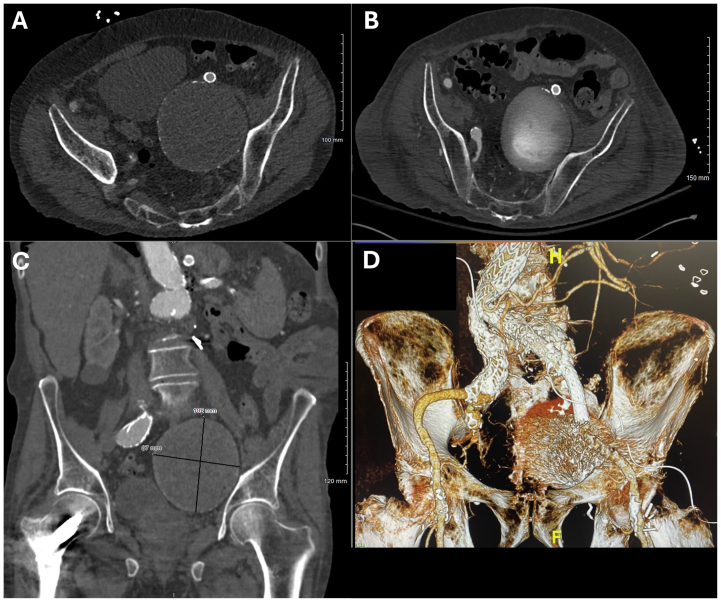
(A-D) Preoperative computed tomography (CT) imaging of a large left internal iliac artery (IIA) aneurysm after prior endovascular aortic aneurysm repair (EVAR). (A) Axial CT scan showing markedly enlarged left IIA aneurysm. (B) CT angiography (CTA) with contrast, consistent with a type II endoleak. (C) Coronal CTA demonstrating the aneurysm and retrograde filling. (D) Three-dimensional reconstruction.
